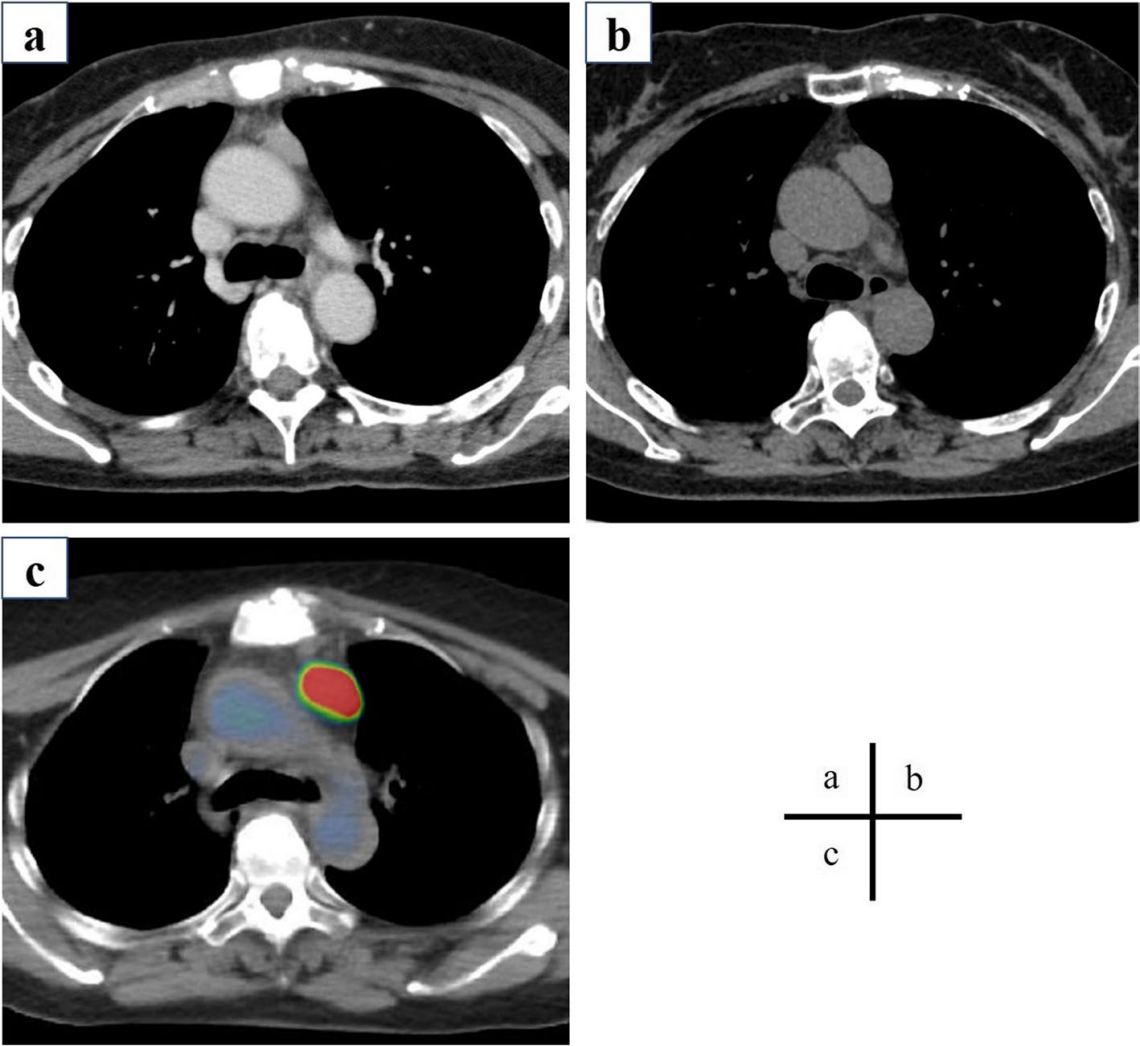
Chest computed tomography revealed a tumor in the anterior mediastinum (a). The tumor enlarged over a period of 2 years (b). PET-CT imaging revealed an FDG accumulation in the tumor (c). PET-CT positron emission tomography with computed tomography, FDG fluorodeoxyglucose
